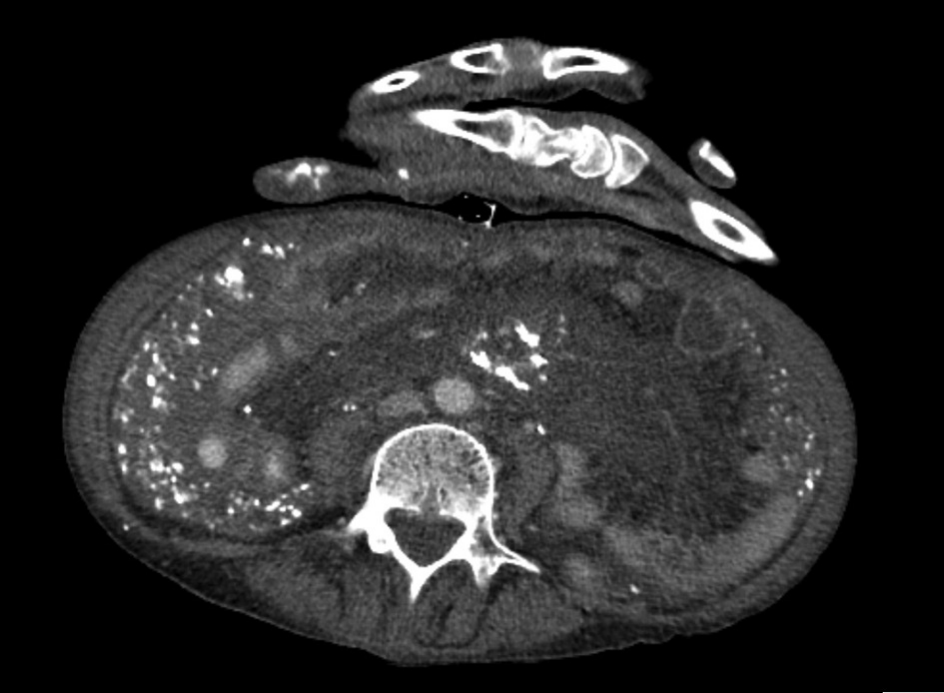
Axial CT scan of the abdomen/pelvis showing “omental cake” with calcified deposits.
